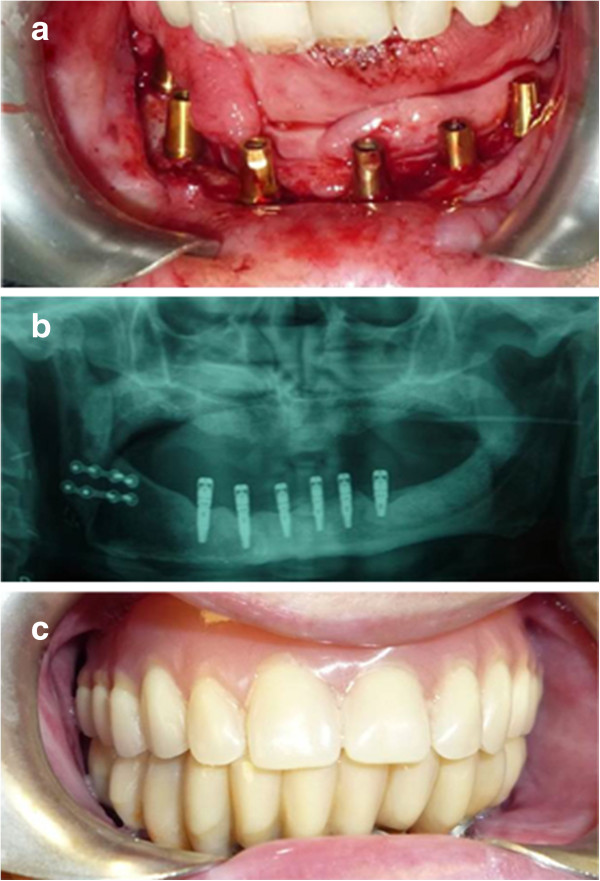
Patient after implant-supported prosthesis rehabilitation. a) implants placed in the jaw after revasculated flap reconstruction, b) final panoramic radiograph with ossointegrated implants, c) implant-supported prosthesis.
